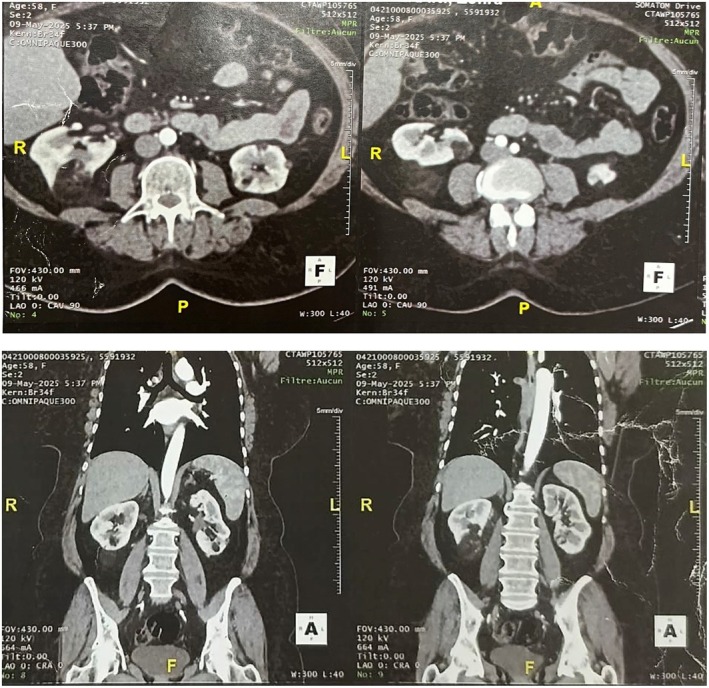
Contrast‐enhanced abdominal CT scan showing a large right renal angiomyolipoma with macroscopic fat and enhancing vascular components.
